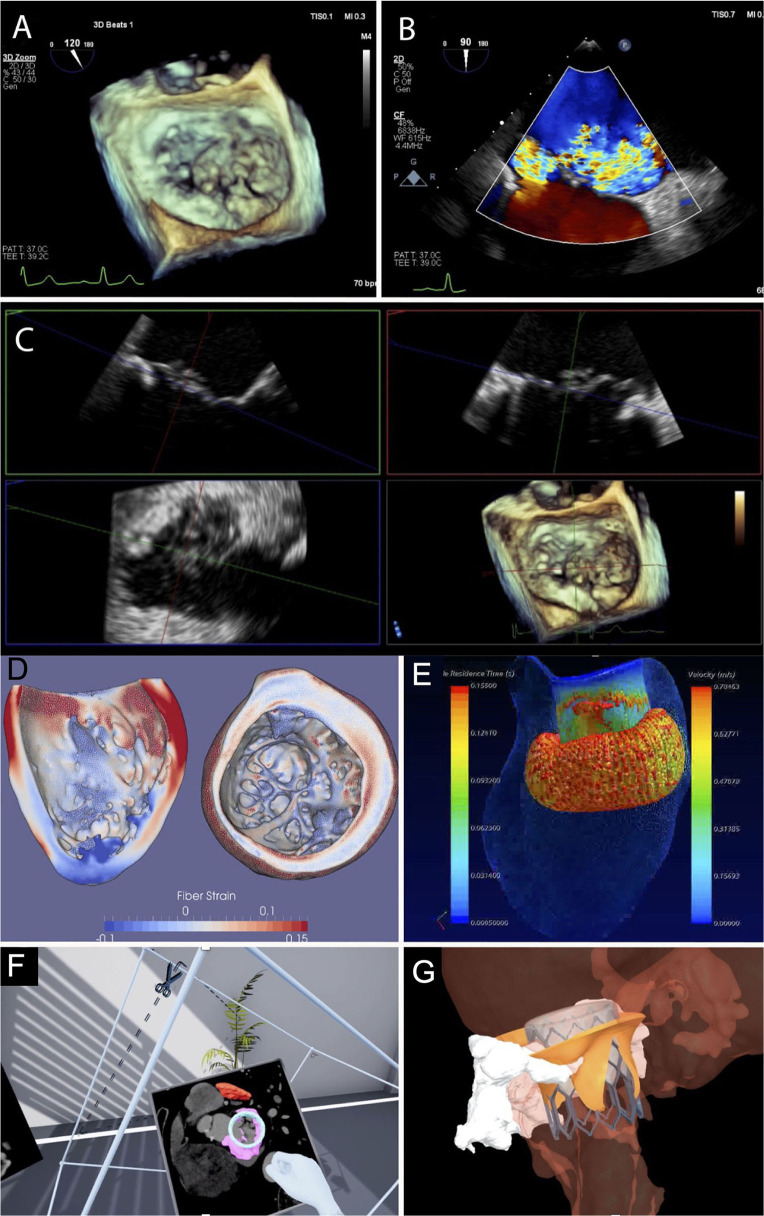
Transoesophageal echocardiogram for edge-edge mitral valve leaflet repair. (A) Shows 3D imaging, (B) Demonstrates Doppler and (C) Dual plane imaging. Advanced imaging processing techniques used within the TMVC. (D) Shows myocardial fiber strain from CT. (E) Demonstrates 4D CT flow to predict blood residence time within the left ventricle following TMVR device deployment. (F) Is an example of virtual reality. (G) Demonstrates virtual implantation and modeling. 3D, 3-dimensional; TMVC, transcatheter mitral valve clinic; CT, computed tomography; TMVR, transcatheter mitral valve replacement.
